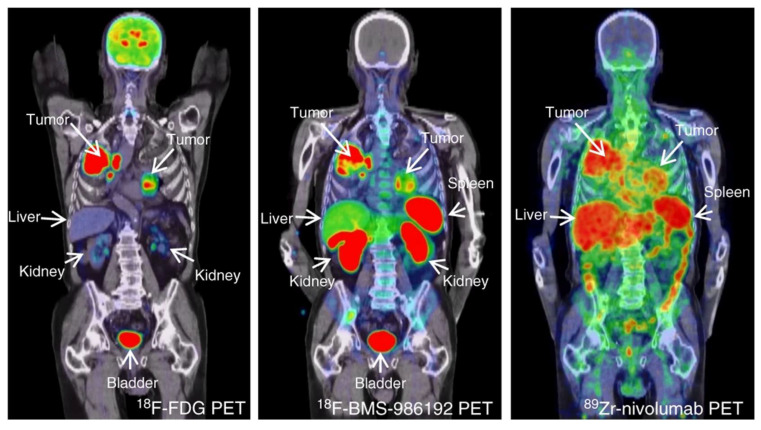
PET imaging of a patient with non-small-cell lung cancer [18]. (Left): Image showing the [^18^F]FDG PET scan, which shows the high glucose metabolism of tumors in both lungs and mediastinal lymph nodes. (Middle): [^18^F]F-BMS-986192 PET (145.7 MBq, 1 h post-injection (p.i.)) and (Right): ^89^Zr-labeled nivolumab PET (37.09 MBq, 162 h p.i.) demonstrate the heterogeneous tracer uptake within and between tumors. The figure is reproduced with permission from [18].
